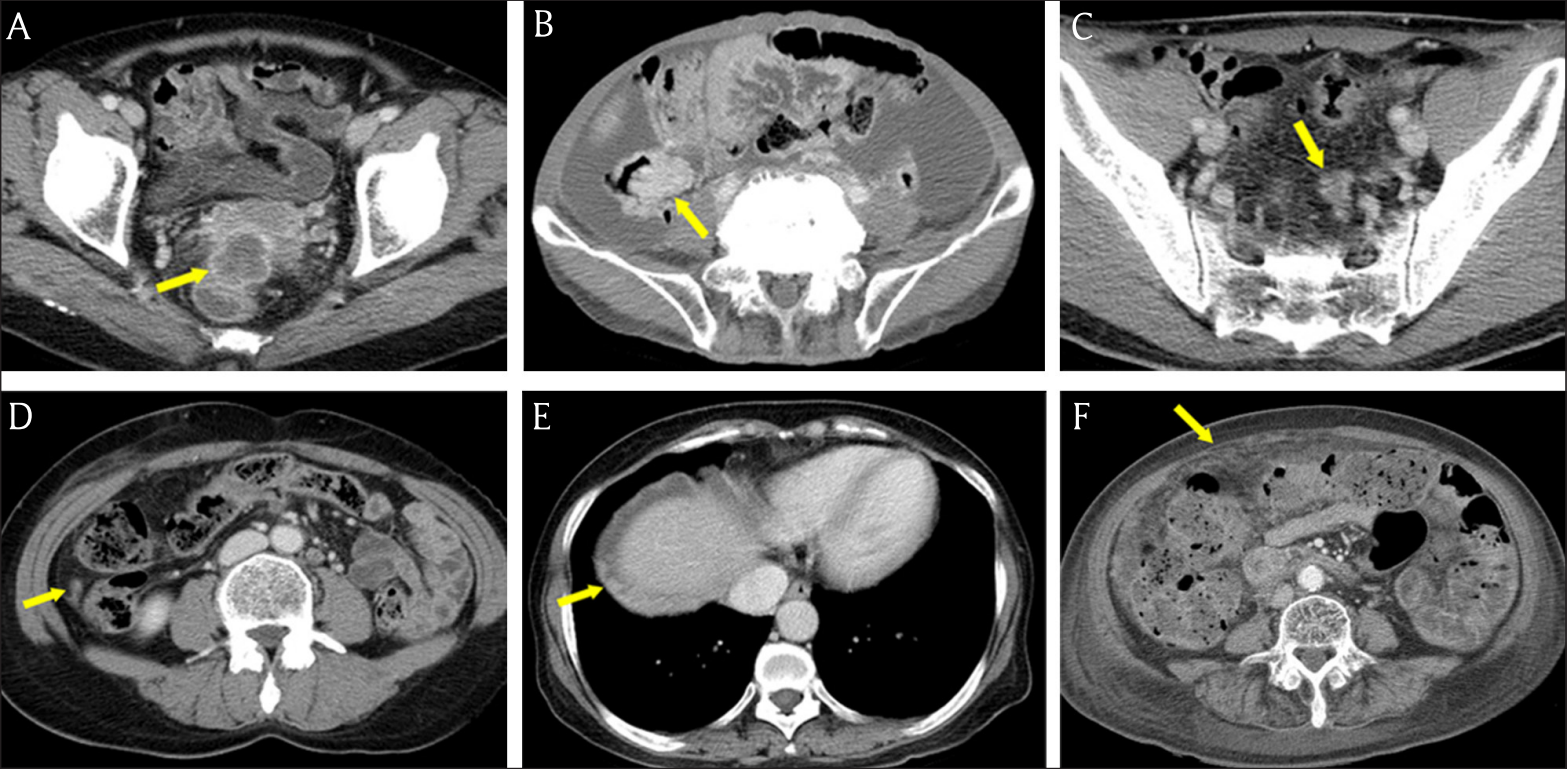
Common seeding sites in peritoneal carcinomatosis. (A–E) Axial portal venous phase CT images show the frequent locations for peritoneal seeding (arrows): peritoneal reflexion (A), lower small bowel mesentery (B), sigmoid mesocolon (C), right paracolic gutter (D), right subphrenic space (E), and greater omentum (F).
